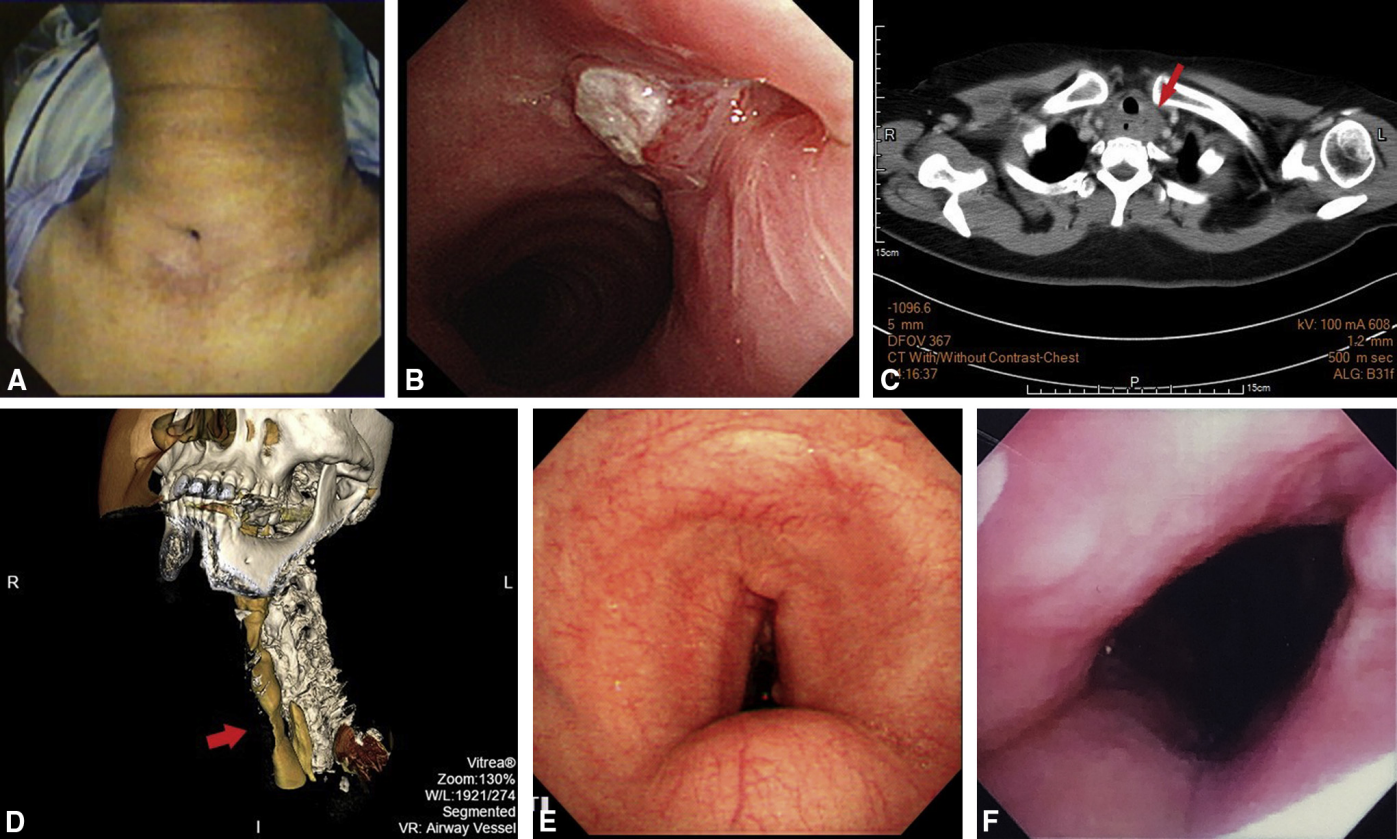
Several indications for the use of a nail graft for tracheal reconstruction. A, The patient had a persistent tracheocutaneous fistula after tracheostomy decannulation. B, Bronchoscopy reveals a persistent tracheocutaneous fistula. C, The patient had recurrent neck lymph node metastasis leading to tracheal compression (*red arrow*) after chemotherapy for lung adenocarcinoma. D, Preoperative 3-dimensional reconstruction CT shows tracheal stenosis (*red arrow*). E, Preoperative bronchoscopy of the fourth patient shows narrowing of the tracheal lumen with associated loss of the semicircular shape and granulation from previous tracheostoma defects. F, At 1 month postoperatively, bronchoscopy of the fourth patient shows improvement of postintubation stenosis.
